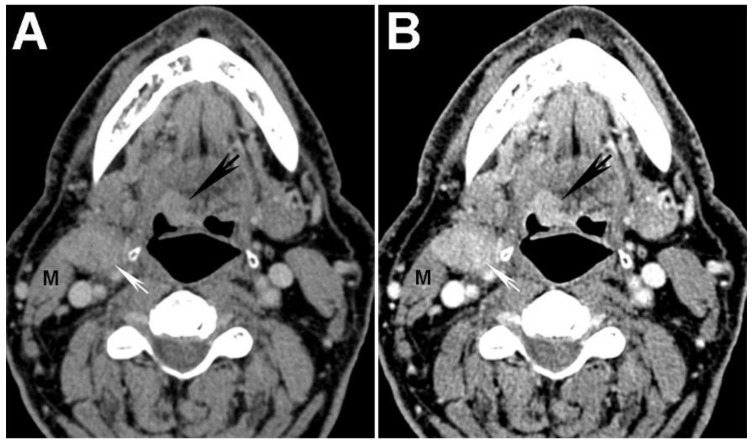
Increased tumor attenuation on 40 keV virtual monochromatic images (VMIs). (A) 70 keV single energy equivalent CT image of a right base of tongue tumor (large black arrow) and pathologic right level IIA lymph node (small white arrow) is shown. Note the similar density of both lesions compared to the normal right sternocleidomastoid muscle (M); (B) On the 40 keV image displayed using the same window-level settings, note the higher lesion density as well as higher relative contrast compared to muscle (M). Also note the increased image noise on the 40 keV VMI (B) compared to 70 keV VMI (A).
